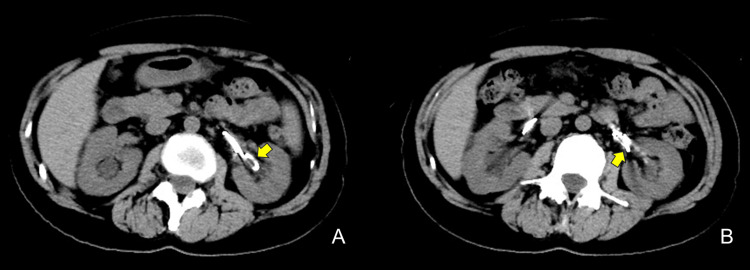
Non-contrast computed tomography (CT), axial images. A: Polyurethane double-J (DJ) ureteral stent (arrow). B: Metallic ureteral stent (arrow).
